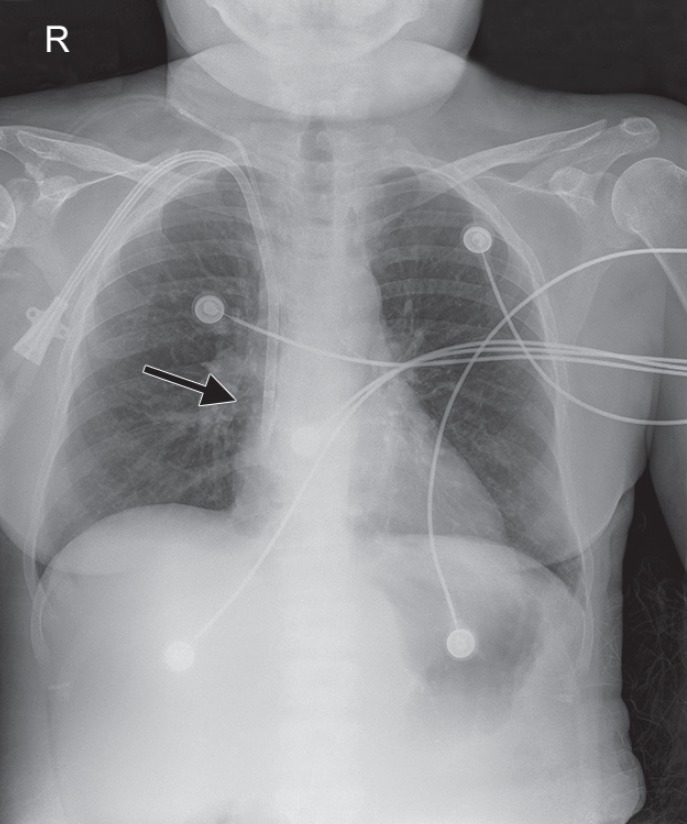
Chest X-ray post right internal jugular CVC insertion demonstrating proximity to right internal jugular dialysis catheter
